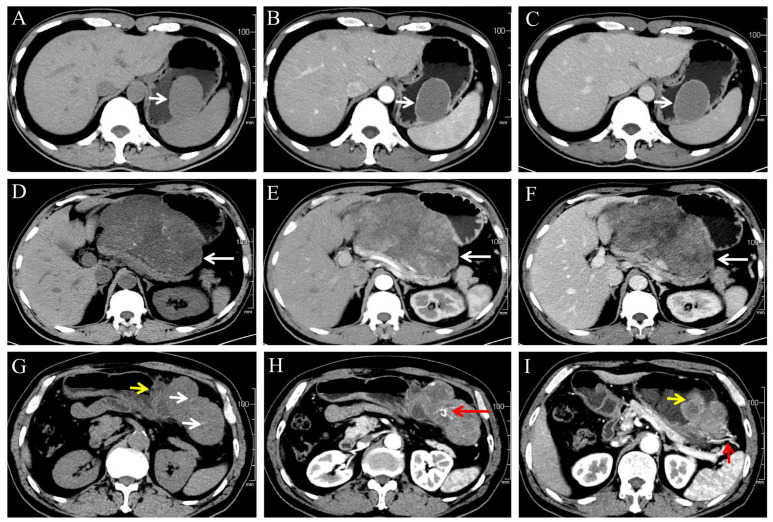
(A–C) A 46-year-old male patient with very low-risk GSTs in gastric body (white arrow). The non-contrast (A), arterial phase (B), and venous phase (C) CT images showed endophytic growth tumor with regular morphology, homogeneous enhancement and no necrosis. (D–F) A 59-year-old male patient with moderate-risk GSTs in gastric body (white arrow). The non-contrast (D), arterial phase (E), and venous phase (F) CT images showed exophytic growth lesion with irregular morphology, thin dotted calcification (D), heterogeneous enhancement, and necrosis. (G–I) A 57-year-old male patient with high-risk GSTs in gastric fundus. The non-contrast (G), arterial phase (H,I) CT images showed a mixed growth tumor with irregular morphology, thin dotted calcification ((G), white arrow), heterogeneous enhancement and necrosis area, feeding artery ((I), short red arrow), vascular-like enhancement ((H), long red arrow), and ulceration ((G,I), short yellow arrow).
